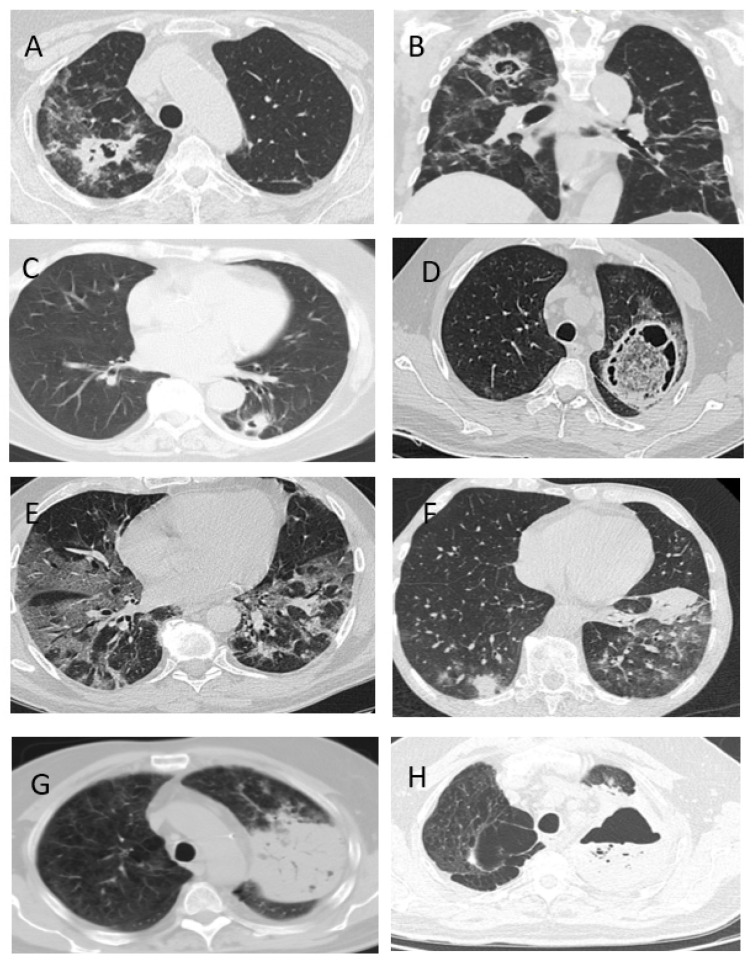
Chest CT scans of the patients with CAPA. (A,B) Patient S., 71 years, with CAPA and decompensated diabetes mellitus. There were cavities of destruction in the upper lobe of the right lung. (C) Patient G., 66 years, with CAPA and rheumatoid arthritis. There were infiltrations with areas of destruction (sickle sign) in S6 of the left lung. (D) Patient C, 35 years, with CAPA and diabetes mellitus with ketoacidosis. Large cavity with content localized in S1 + 2 of the left lung. (E) Patient K., 62 years, with CAPA and the debut of diabetes mellitus. There were multiple bilateral infiltrations with “frosted glass” sites and areas of consolidation. (F) Patient J., 68 years, with CAPA and multiple myeloma out of remission. On CT-scans in both lungs, there were polysegmental foci of “frosted glass” compaction and consolidation areas. (G,H) Patient C., 59 years, with CAPA. On CT-scans that showed increased dynamic infiltration and hydrothorax, destruction appeared in line with the level of fluid.
